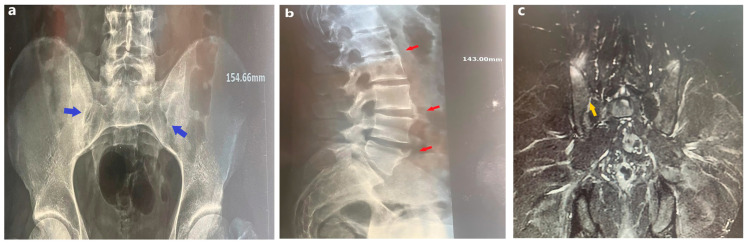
Bilateral sacroiliitis grade III and ankylosing spondylitis—anterior to posterior pelvic radiographic evaluation (a), lateral lumbar spine radiographic evaluation (b) and coronal plane MRI (c). Sacroiliitis with erosions, evidence of sclerosis, and an ill-defined sacroiliac joint (blue and yellow arrows). Syndesmophytes formation with a vertical orientation at the level of L1–L2 and L3–L5 (red arrows).
